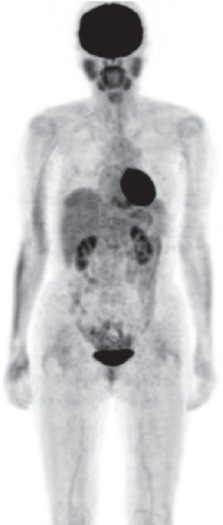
Maximum intensity projection (MIP) image of FDG-PET after the completion of combined chemoimmuno-therapy. Abnormal FDG uptakes completely disappeared
